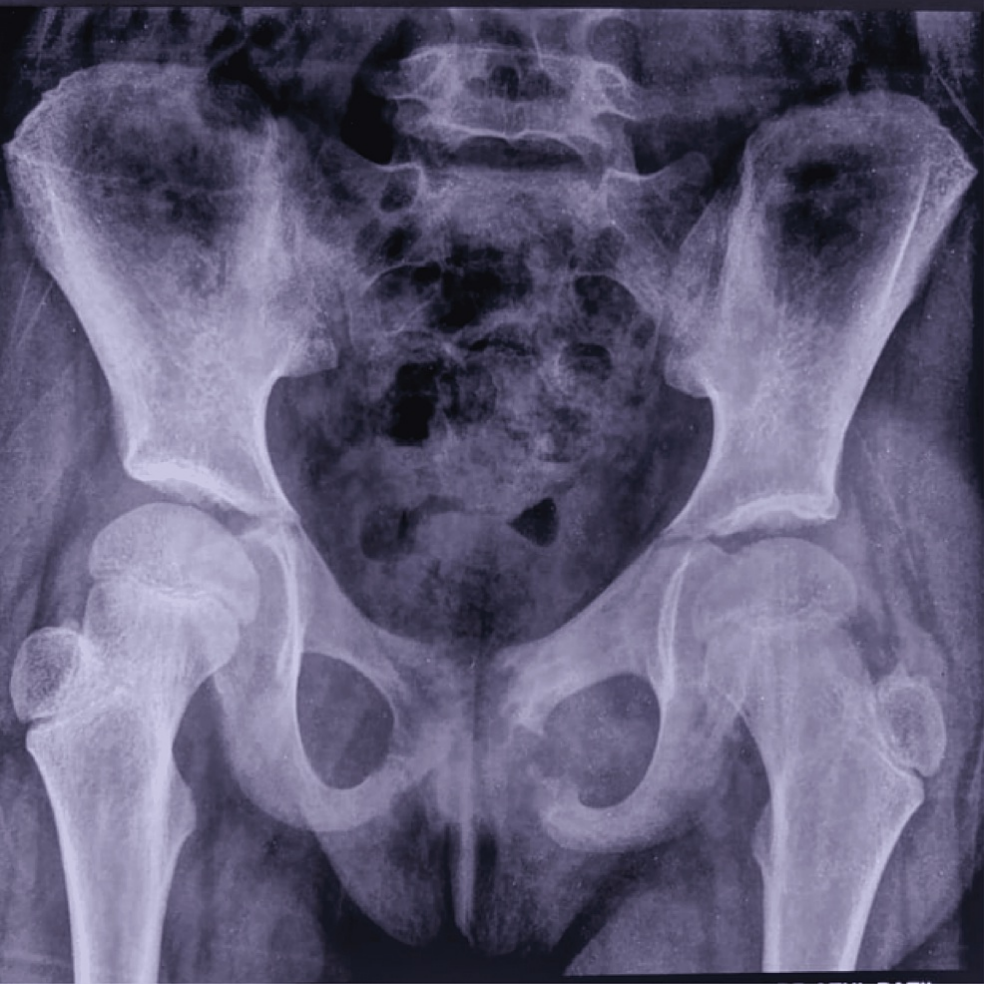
X-Ray of pelvis revealing bony erosion of pubic symphysis
